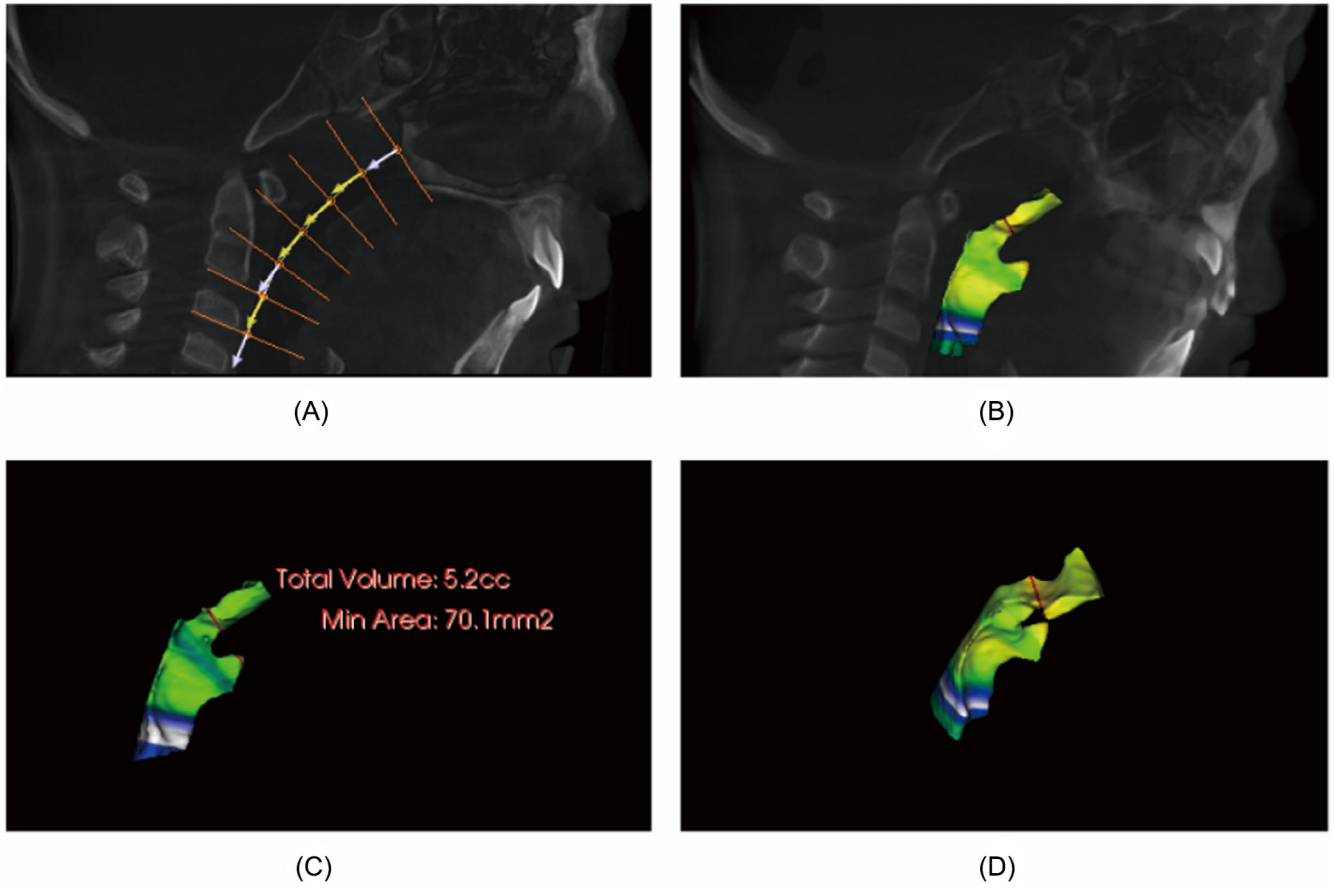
3D airway reconstruction by CBCT. (A) The area between the parietal wall of the nasopharynx and the inferior border of the 3rd cervical vertebra was selected for airway localization. (B) 3D airway angioplasty was performed at the end of localization. (C) Bone and soft tissue artifacts were removed to show the total airway volume and the minimum cross‐sectional area of the posterior nasal aperture with airway compression. (D) The airway was rotated at a certain angle after 3D imaging to show the obvious airway compression and the minimum cross‐section (marked in red).
